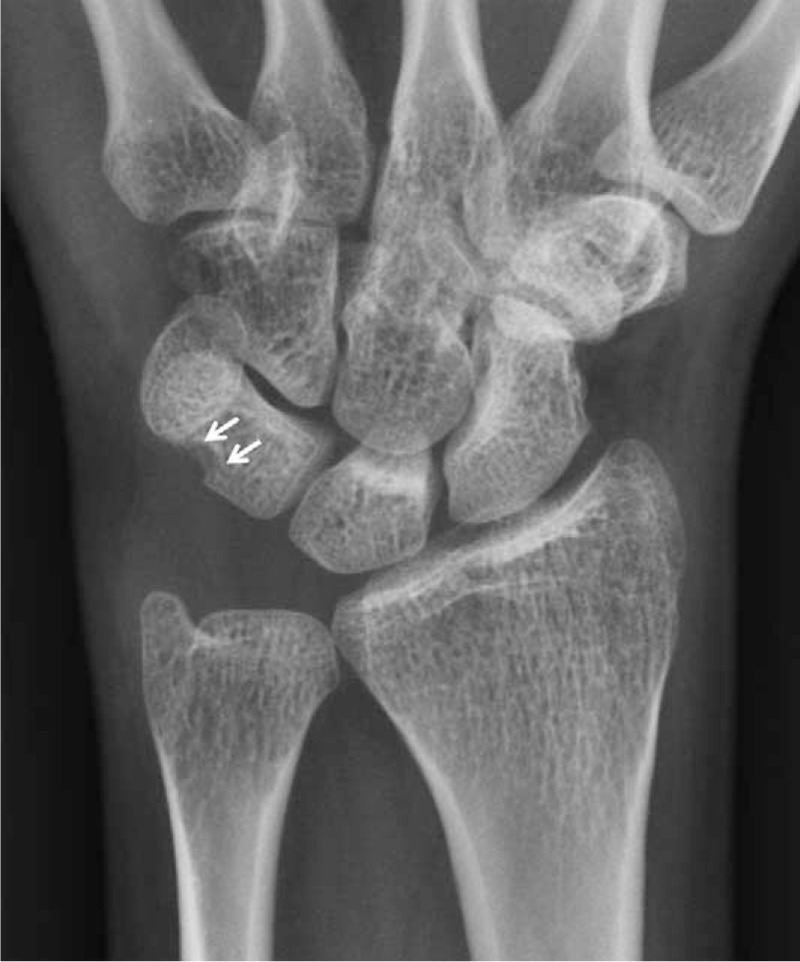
Anteroposterior radiograph of the left wrist, showing pressure bone erosion with sclerotic margin in the triquetrum (arrows).
